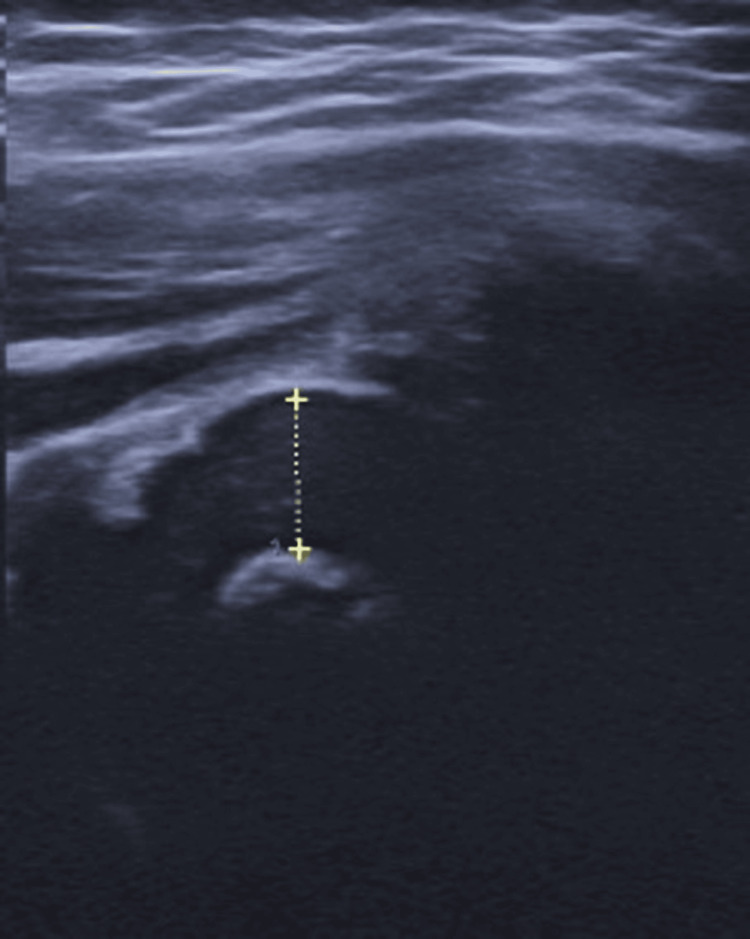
Ultrasound scan of the right hip showing fluid (yellow dots) in the hip joint
